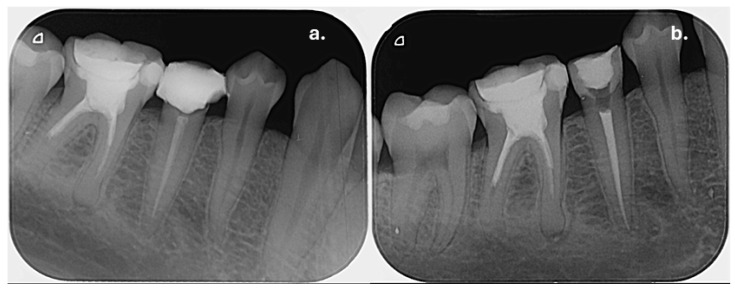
(a) Diagnostic periapical radiograph of tooth 45; (b) Periapical radiograph following the root canal treatment of tooth 45.
